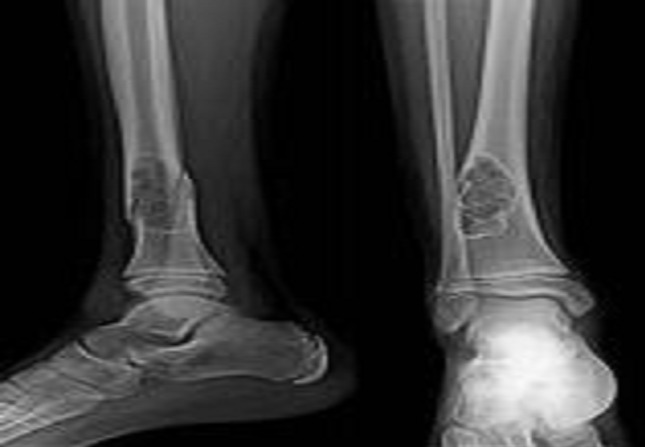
Radiograph showing an osteolytic lesion well demarcated from the lower end of the tibia containing the partitions associated with a spiral fracture
